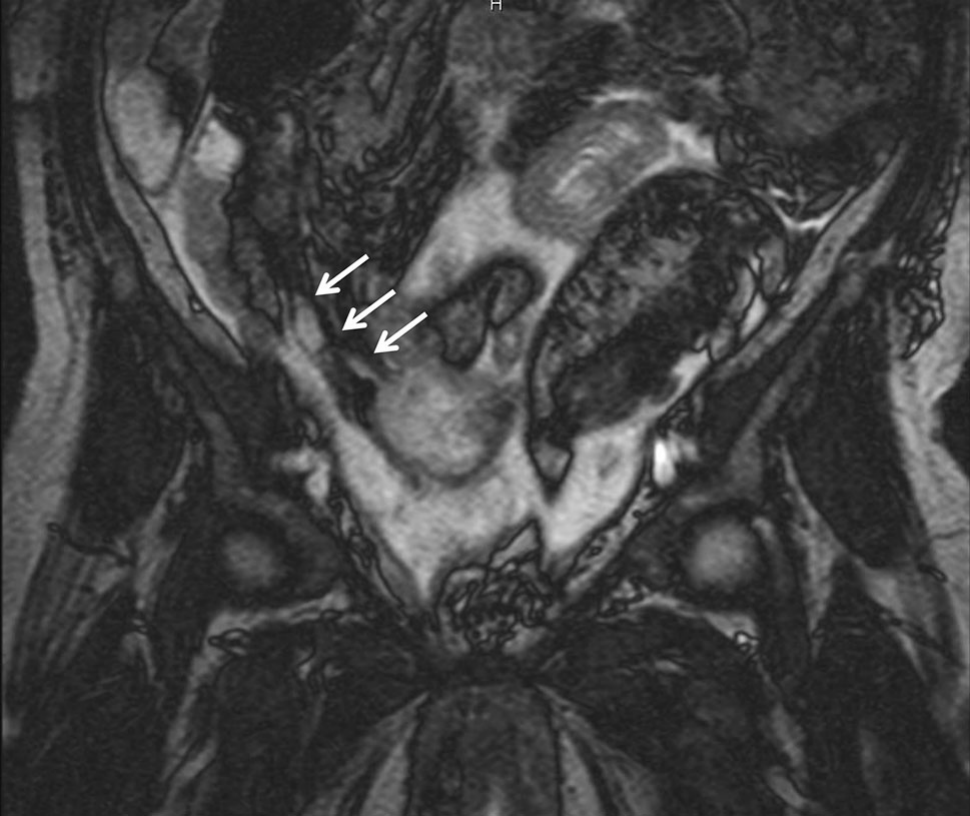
Magnetic resonance imaging (MRI) findings. A cystic tumor is arising from the appendix
